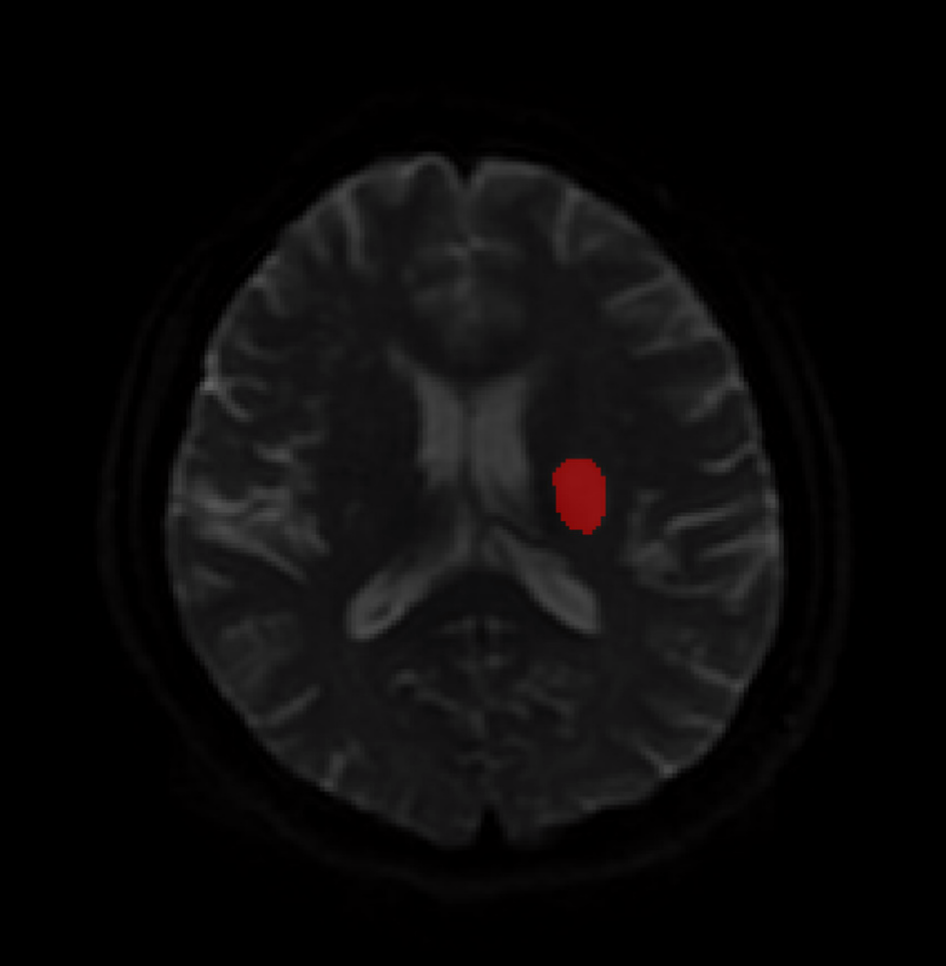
An example of infarct volume views on diffusion-weighted imaging (DWI).
